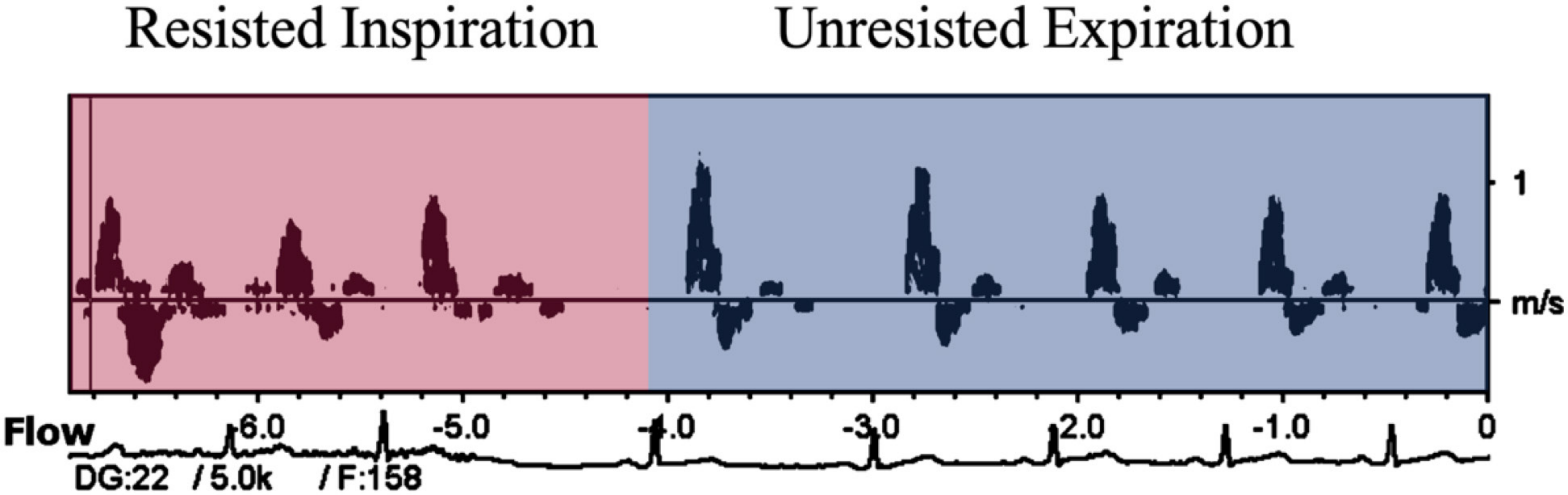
Ultrasound doppler of blood velocity during resisted inspiration (pink) and unresisted expiration (blue).
